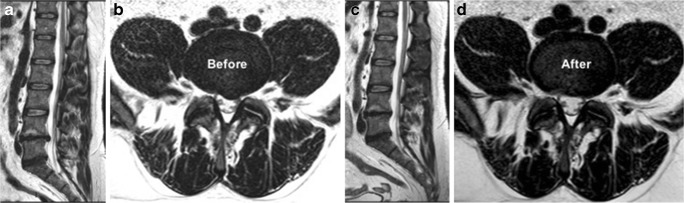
MR images of a 71-year- old man complaining of left lumbosciatica of 2-year duration and showed a significant clinical and radiological improvement after intradiscal O_2_-O_3_ injection. a, b Preprocedure sagittal and axial MRI T2-weighted images showing an extruded left para-centeral disc herniation at the level of L4–5, compressing the dural sac and the descending nerve roots. c, d Follow-up sagittal and axial MRI images obtained 6 months after the procedure showing near-complete resolution of the extruded disc hernia
